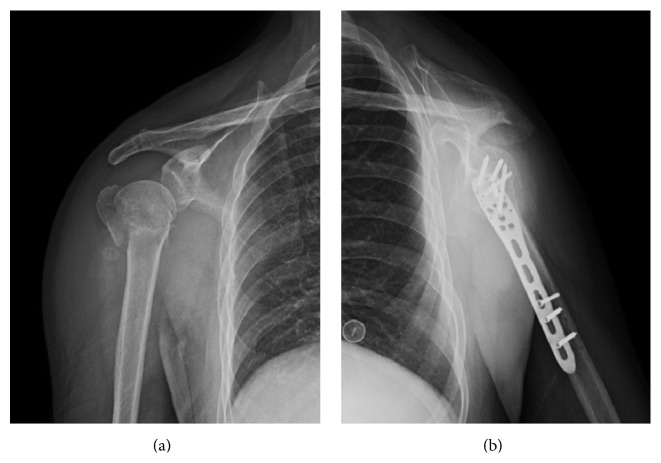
Preoperative (a) and postoperative (b) radiograph (lateral view) of proximal humeral fractures in a patient with II-part greater tuberosity fracture.
